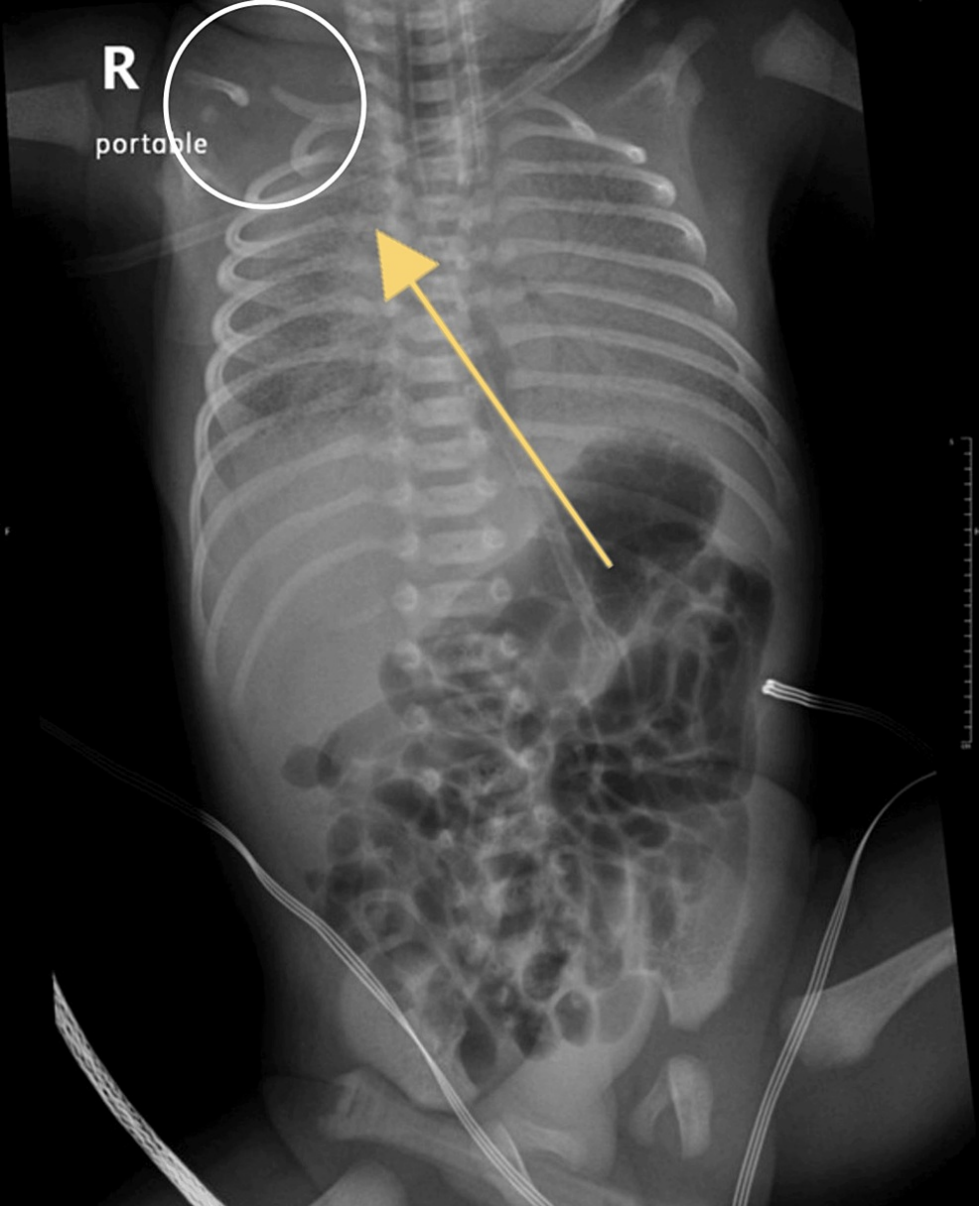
Chest X-ray of the first case showing the deformity of the clavicle on the right side.
